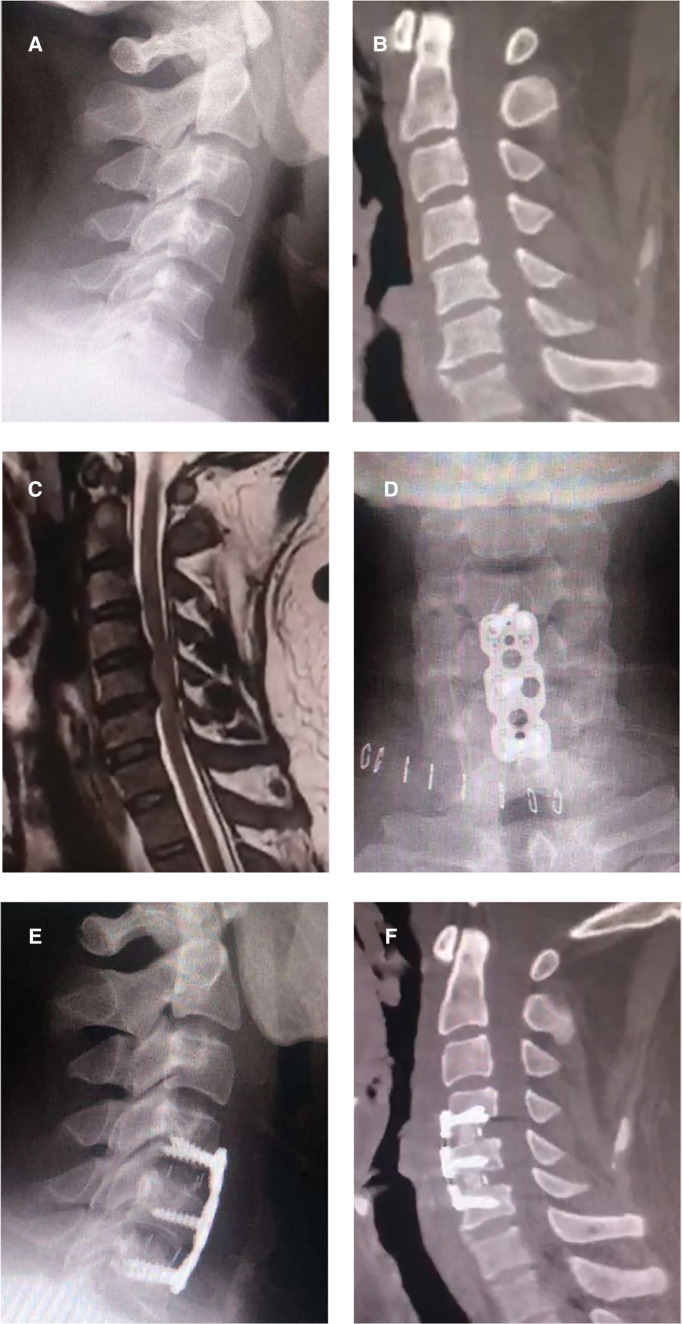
Preoperative and postoperative images of anterior cervical discectomy and fusion. (A) A preoperative lateral x-ray image of the patient; (B) A preoperative sagittal CT image of the patient; (C) A preoperative sagittal MR image, revealing spinal stenosis at the C4/5 and C5/6 levels secondary to central disc herniation; (D–F) Postoperative x-ray and CT images, revealing accurate placement of the plates, screws, and cages.
